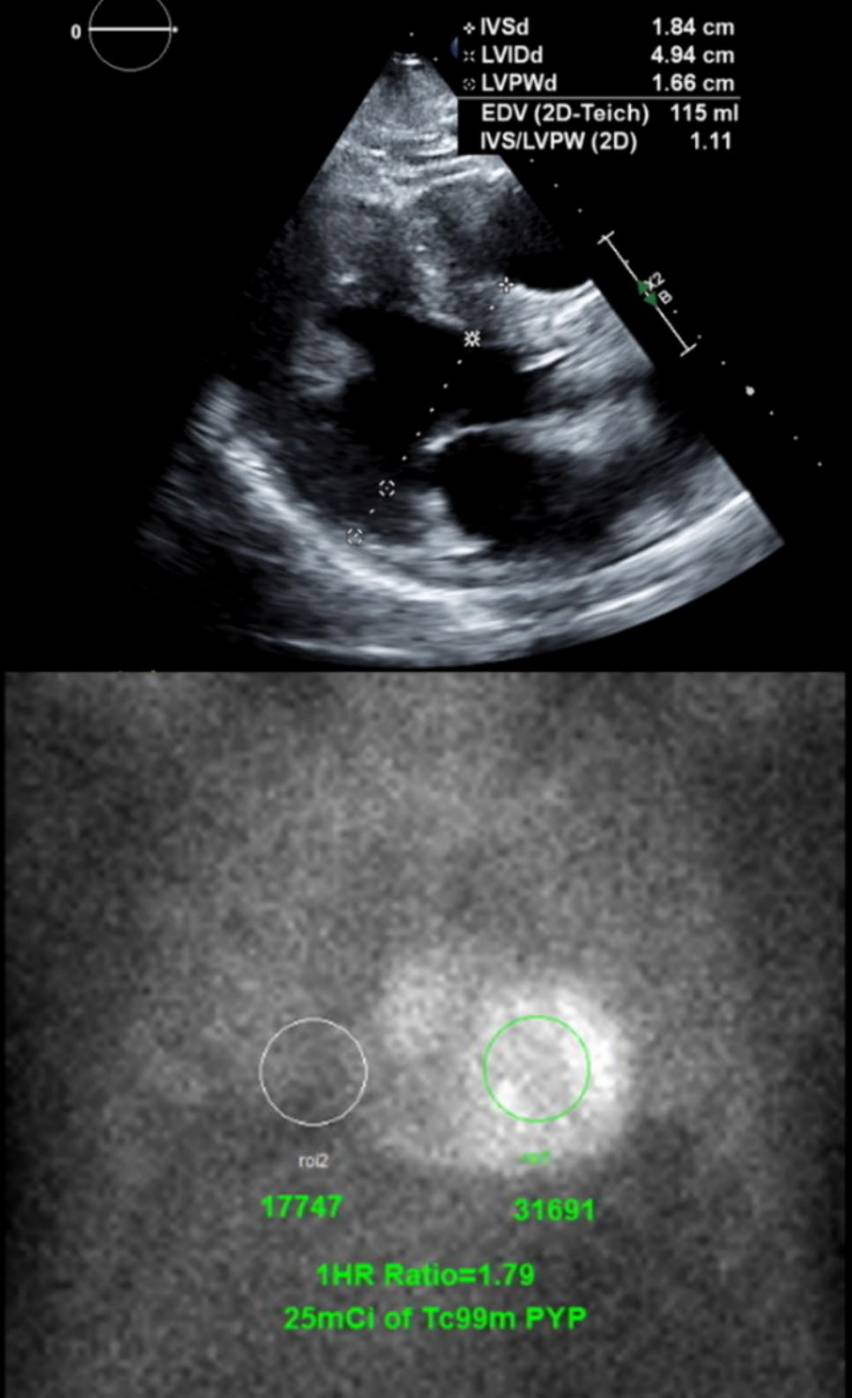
Suspected Transthyretin Cardiac Amyloidosis Diagnosed by ^99m^Tc-pyrophosphate Scintigraphy. (Top) Patient with new heart failure and severe concentric hypertrophy parasternal long-axis view on echocardiography. (Bottom) ^99m^Tc-pyrophosphate scintigraphy showed increase myocardial uptake compared to contralateral chest with a ratio of 1.79, consistent with transthyretin cardiac amyloidosis
